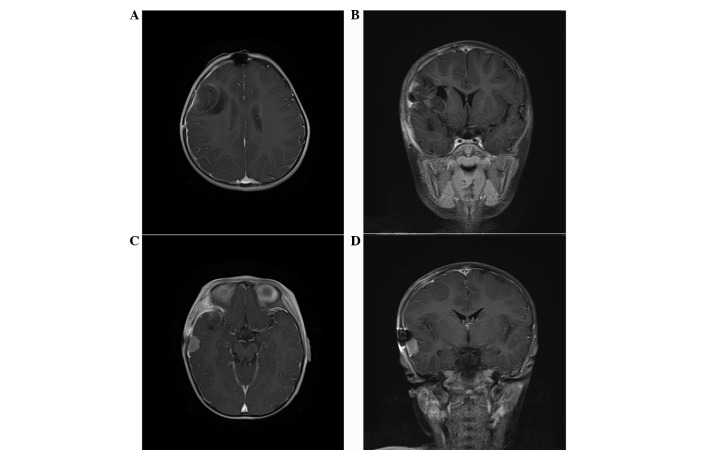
MRI scan with enhancement performed 10 weeks after the initial resection, showing an enlargement of the two tumor nodes identified six weeks previously. (A and C) Axial and (B and D) coronal images were captured. Notably, the tumor node located in the initial surgery field showed mild enhancement, but the other tumor node located in the area posterior-inferior to the initial surgery field showed significant enhancement. MRI, magnetic resonance imaging.
